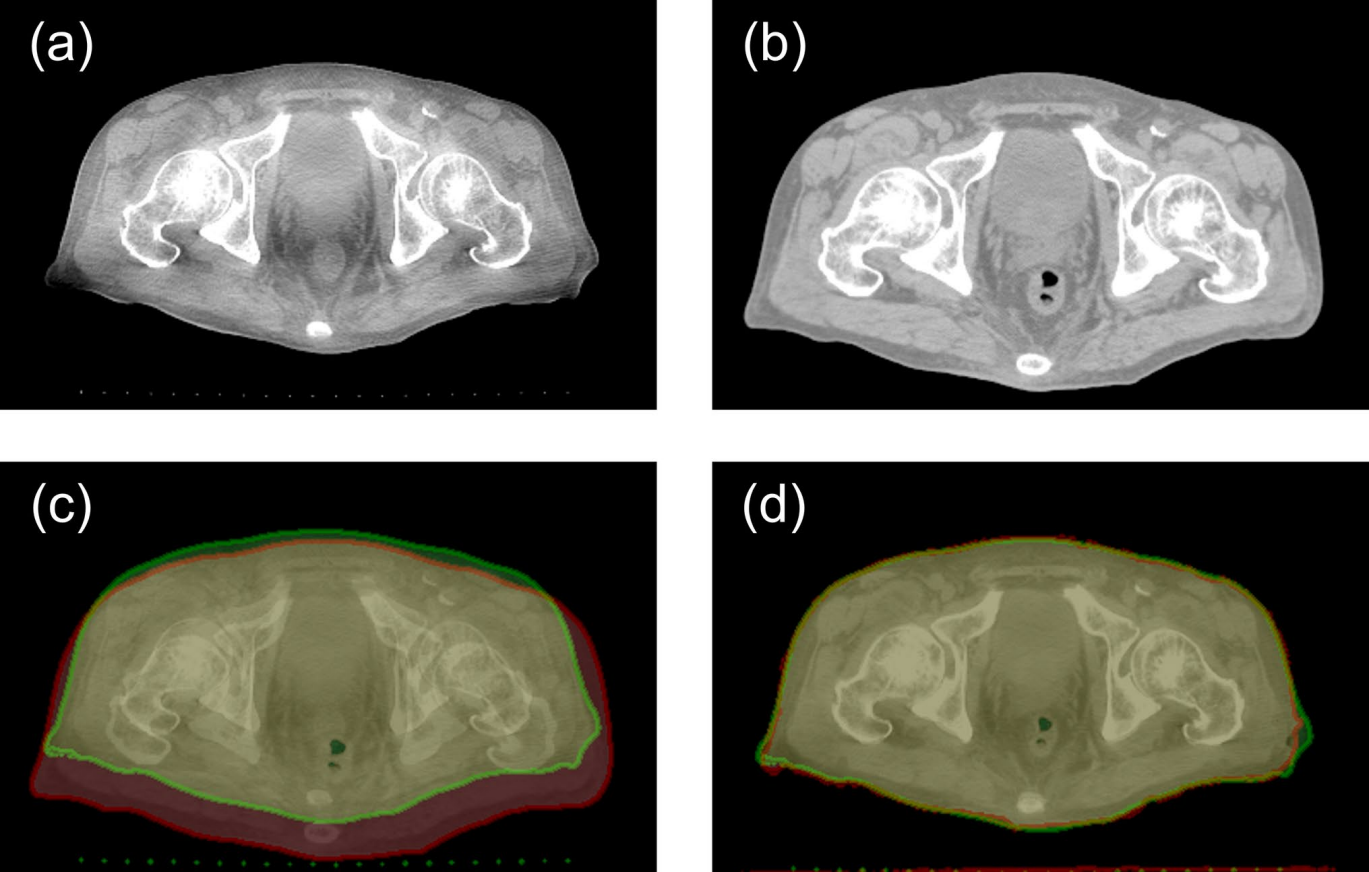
Results of superimposition of treatment planning CT images on CBCT images by B-spline deformable registration: CBCT image as a low-resolution image (a), treatment planning CT image as a high-resolution image (b), alpha blending image of CBCT image (green) and treatment planning CT image (red) before registration (c), and alpha blending image after registration (d)
